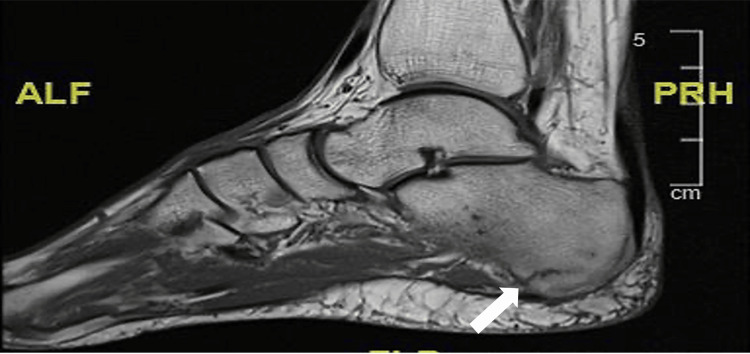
Original T1-weighted MRI of the patient's left ankle. Incomplete banding of low signal in the plantar cortex of the posterior calcaneus, consistent with bone stress injury, highlighted with white arrow. MRI: magnetic resonance imaging; ALF: anterior lateral foot; PRH: posterior hindfoot
